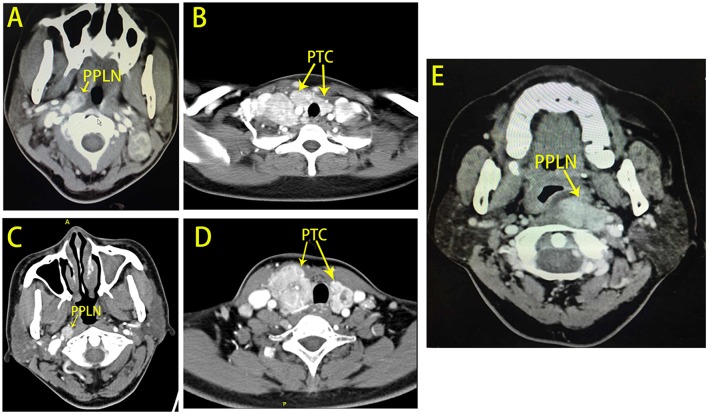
Computed tomography (CT) scans for patients with PPLN metastases. (A,B) Show patient No. 3 with bilateral papillary thyroid carcinoma with right PPLN metastases. (C,D) Show patient No. 4 with bilateral papillary thyroid carcinoma with right PPLN metastases. (E) Shows patient No. 5 with left PPLN recurrence 17 years after the first surgery.
